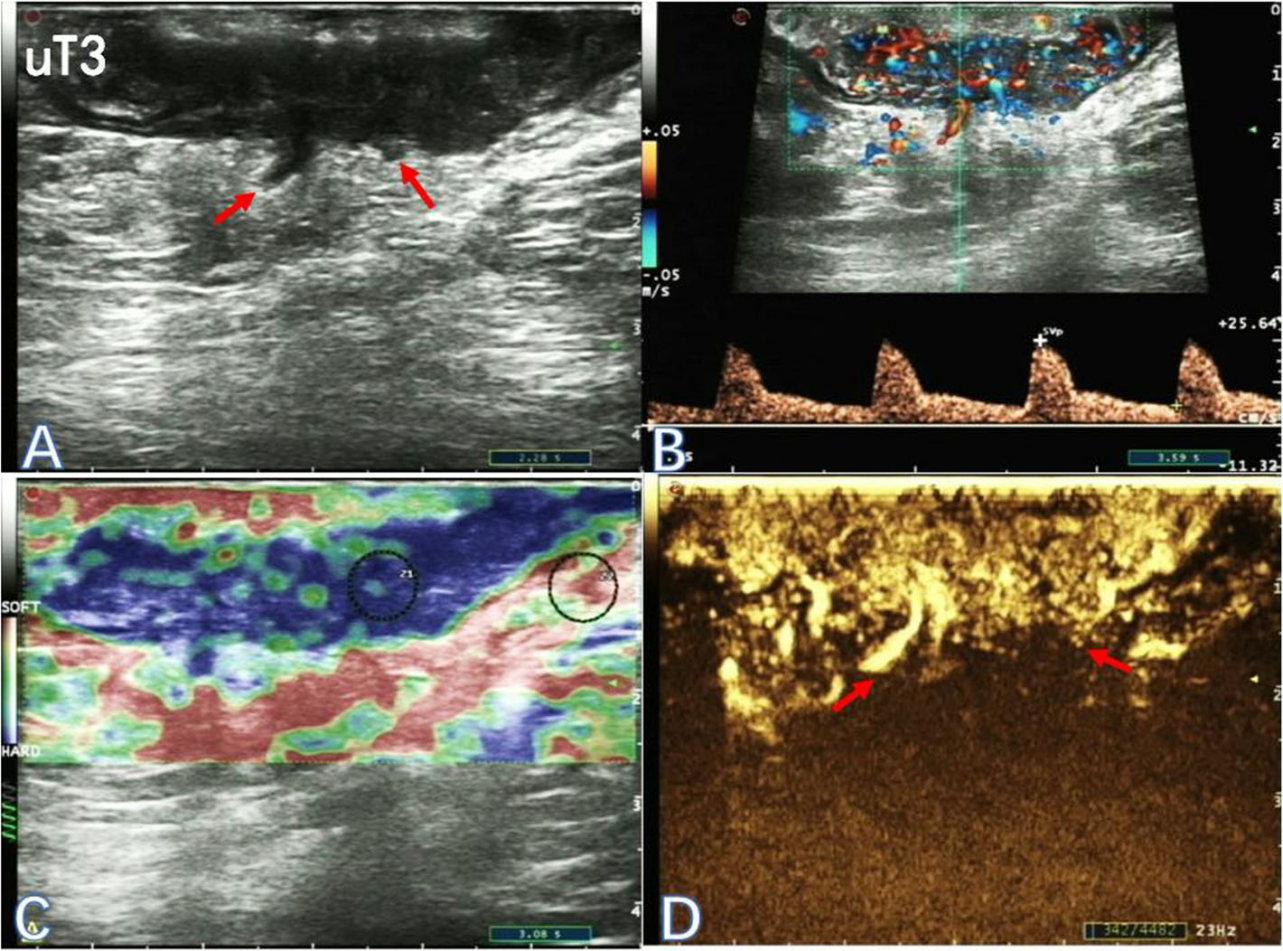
Two-dimensional ultrasonogram (a), color-flow and pulsed Doppler image (b), elastogram (c) and the corresponding contrast-enhanced ultrasonogram (d) of a 61-year-old male patient with stage uT3 low rectal cancer (red arrow)
